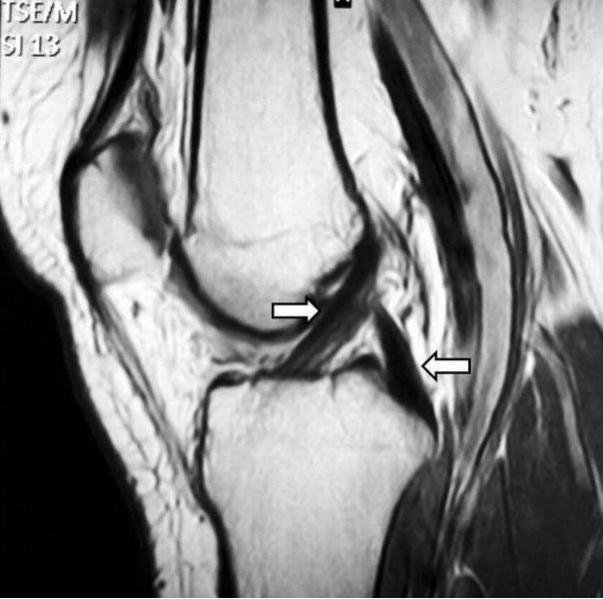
Magnetic resonance imaging evaluation demonstrated normal signal of both anterior and posterior cruciate ligaments (arrows).
